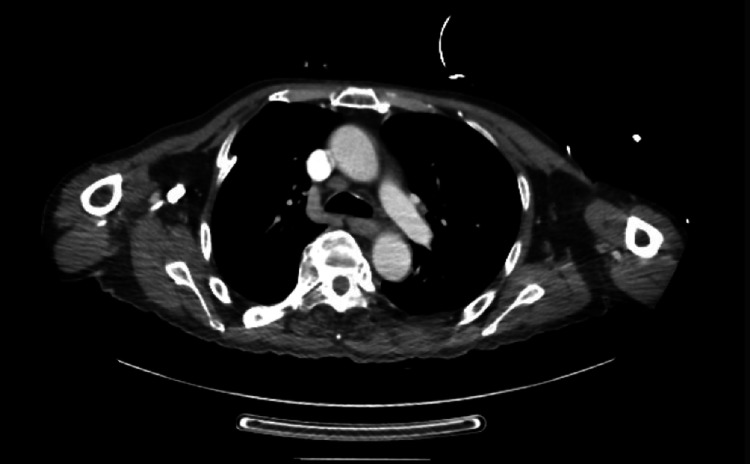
CT of the chest with contrast. Diffuse irregular wall thickening involving the mid- to distal esophagus suspicious for malignancy.
